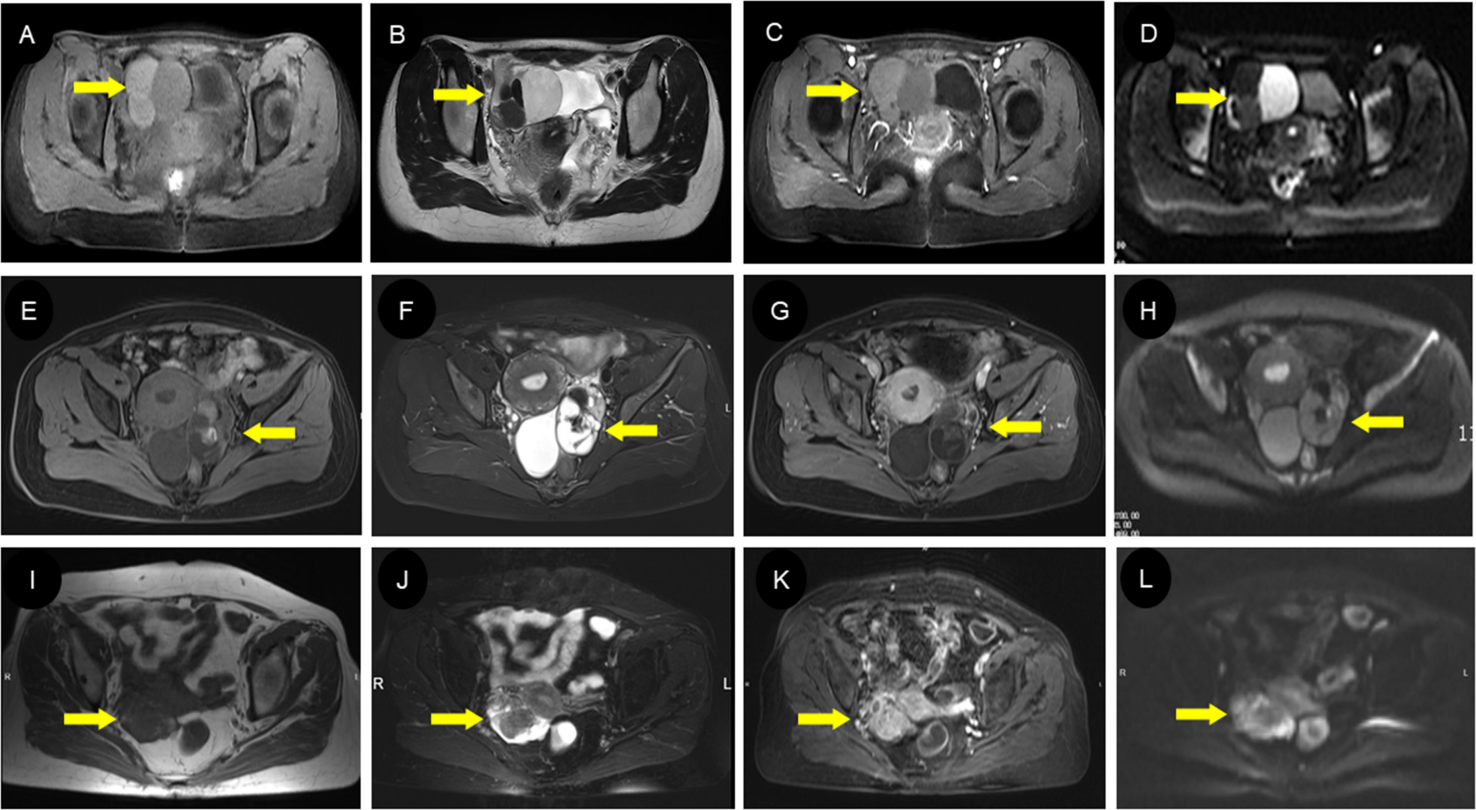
MRI findings of different types of struma ovarii. (A–D) A 23-year-old woman with cystic-type SO with multiple circular signal shadows in the right ovary. The cyst had slightly low signal in axial T1WI (A) and high/low signal in T2WI (B) with enhanced internal septa (C). The DWI images (D) exhibited diverse cyst signals, suggestive of the varying viscosity of the cyst fluid, which can be indicative of a diagnosis of SO. The extremely low signal on T2WI (B) represents the colloidal substances consisting of thyroglobulin, thyroid hormones, and so on, which is of great value in diagnosing SO. (E–H) A 44-year-old woman with mixed-type SO with a solid and cystic mass in the left ovary. There was heterogeneous signal intensity on both T1WI (E) and T2WI (F) owing to regions of fluid, hemorrhage, and calcification; prominent contrast enhancement was seen in the solid part (G). Locally hyperintense regions on the DWI (H) are presented on the DWI images, suggesting a higher concentration of thyroglobulin within the lesion. (I–L) A 71-year-old woman with solid-type SO with a heterogeneous lesion in the right ovary. Most parts of the lesion showed iso-intensity on both T1WI (I) and T2WI (J) with vivid uneven enhancement (K), while the surrounding wreath-like cystic part presented slightly hyperintense signals on T1WI (I), markedly hyperintense signals on T2WI (J), and hyperintense signals on DWI (L). SO, struma ovarii; T1WI, T1-weighted imaging; T2WI, T2-weighted imaging; DWI, diffusion-weighted imaging.
